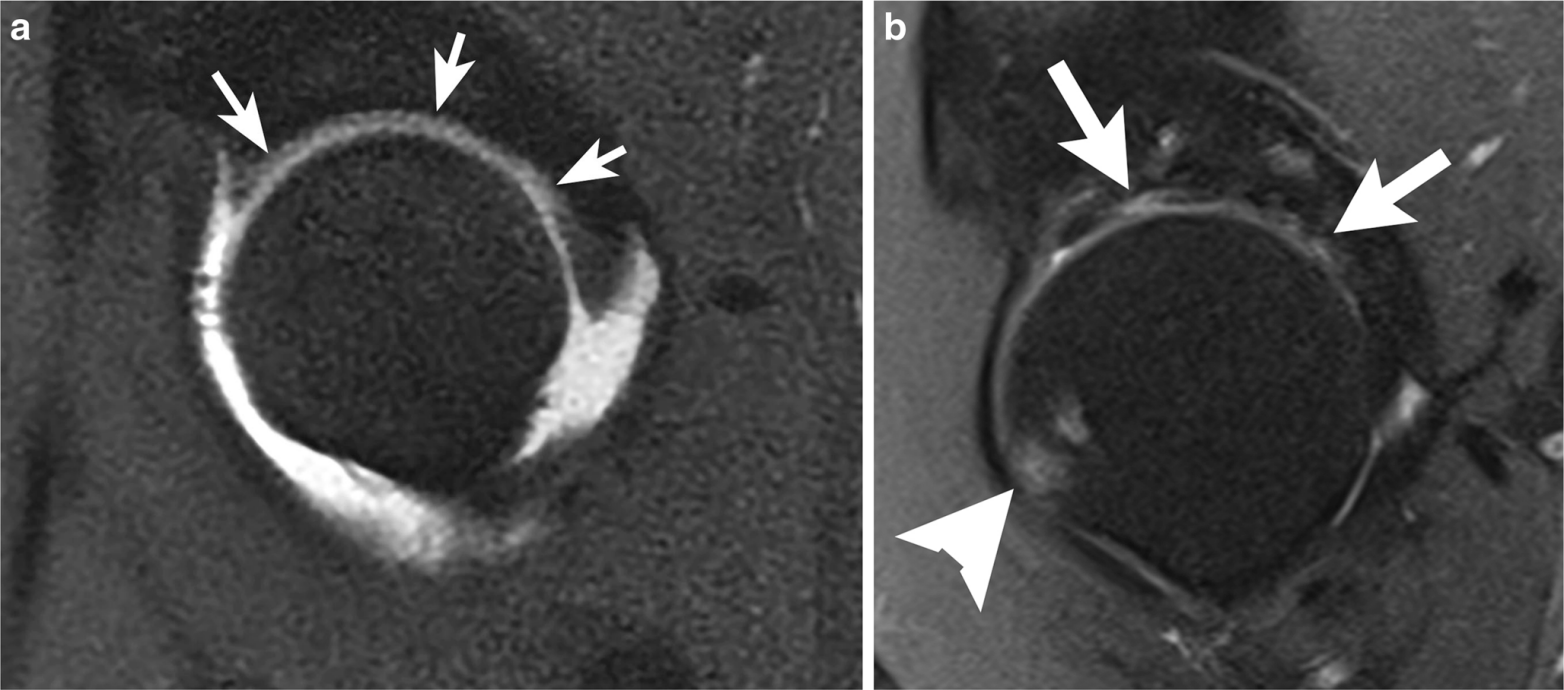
A 30-year-old woman with pain 1 year after arthroscopy. a Preoperative sagittal proton density-weighted fat-saturated (PDFS) MR arthrogram at 1.5 T shows homogeneous, intermediate gray articular cartilage (*arrows*) with a small amount of quantum mottle. b Postoperative sagittal PDFS MRI at 1.5 T shows fluid extending into acetabular cartilage defects (*arrows*), including a small region of cartilage delamination. It is impossible to know on MRI whether this represents progression of disease or iatrogenic damage occurring at the time of arthroscopy.* Arrowhead* points to bone marrow edema in the femoral head, suggesting ongoing damage in this region as well. Edema due to surgery would be expected to resolve in 4–6 months
